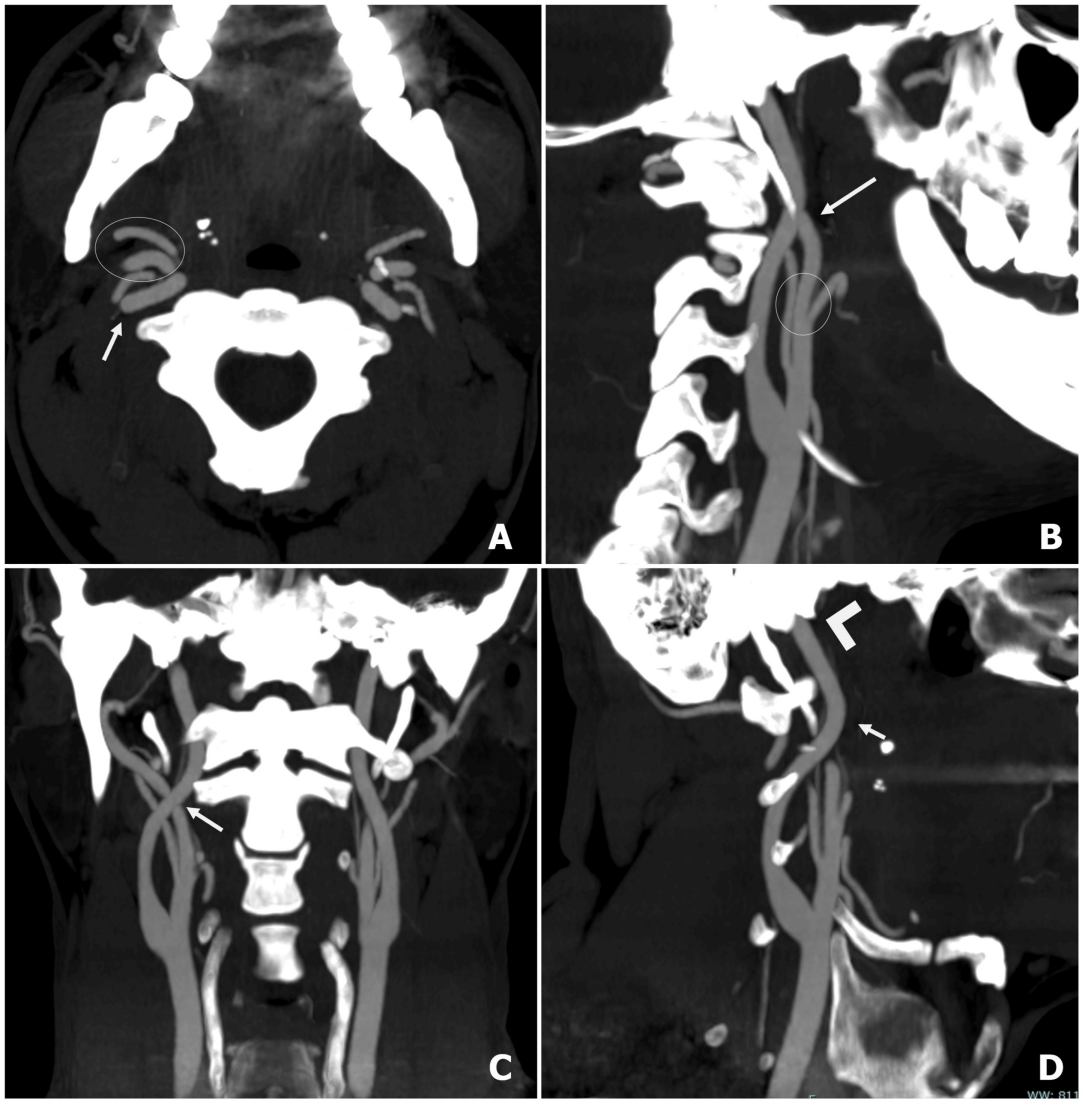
High cervical ICA segment course. Maximum intensity projection (MIP) CT angiography (CTA) shows anteromedial internal carotid artery (ICA) course (white arrow) crossing external carotid artery (ECA) branches (white circle) in axial (A), sagittal (B), coronal (C), and oblique (D) planes. ICA cervical segment ends at the level of the carotid canal (arrowhead in D).
